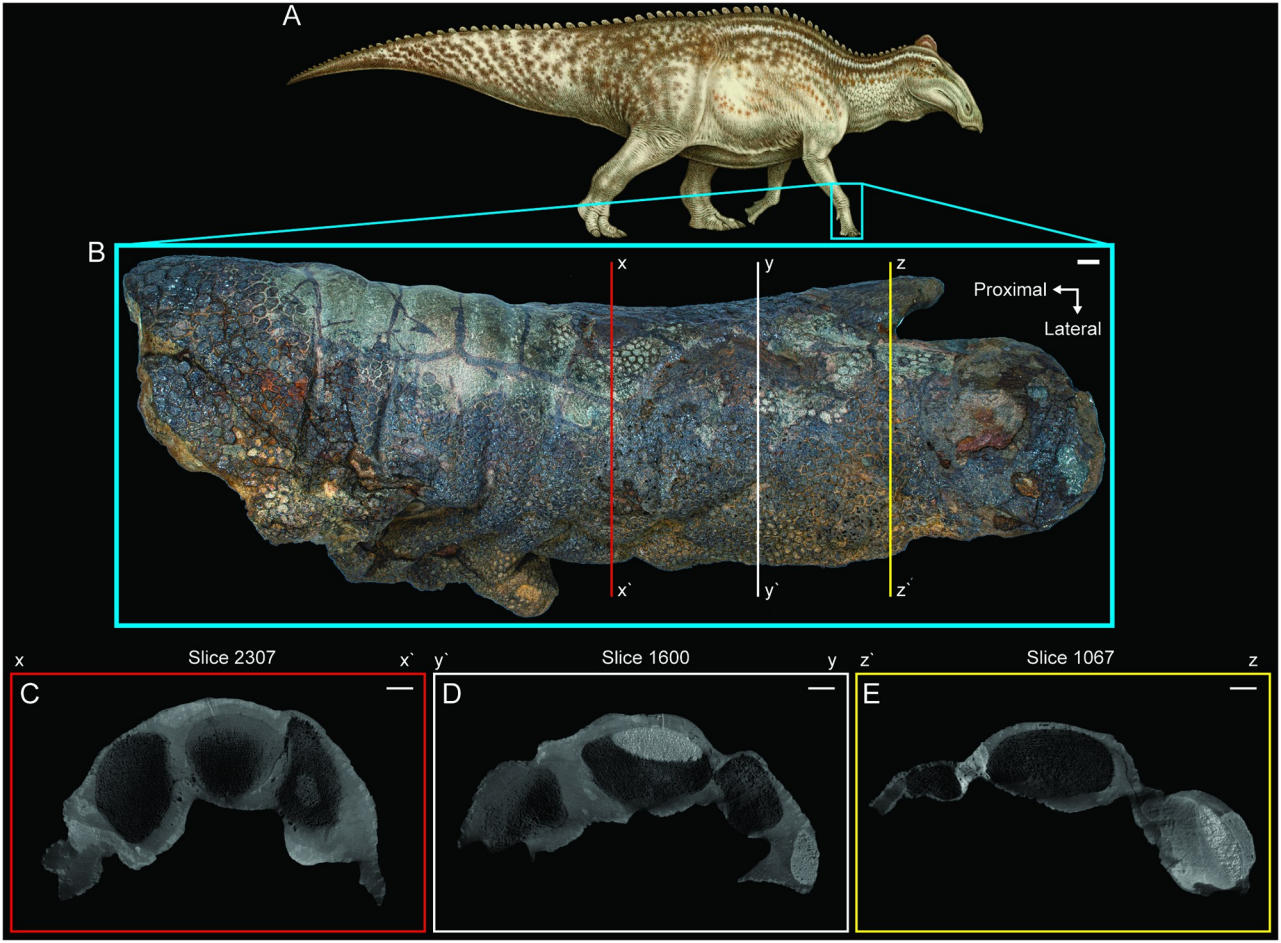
Cross sectional views through the right manus of NDGS 2000. (A) NDGS 2000 reconstruction in right lateral view. (B) Right manus in dorsal view indicating the positions of the three cross sectional views. (C) CT image along line x to x’. (D) CT image along line y to y’. (E) CT image along line z to z’. In (C), (D), and (E), slice numbers from the original CT data are provided above each image. Paleoart in (A) by Natee Puttapipat. Scale bars equal 1 cm.
